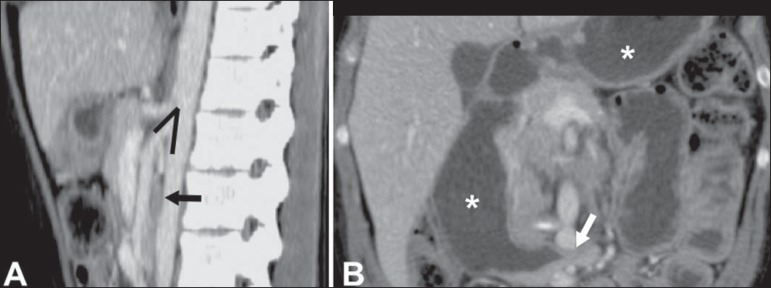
Superior mesenteric artery syndrome. A: Sagittal CT showing
